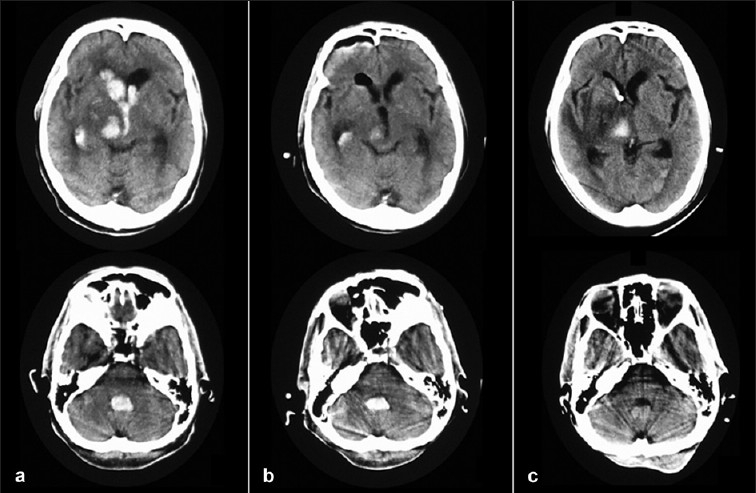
Serial CT scans of patients with thalamic hemorrhage and IVH. The IVH was observed at all the ventricles in the CT scan taken at admission (a). The IVHs at the F. Monro and the Third V. were removed, and the IVH at the fourth ventricle persisted postoperatively (b). The IVH at the fourth ventricle was not observed on the CT scan obtained on postoperative day 6 (c)
